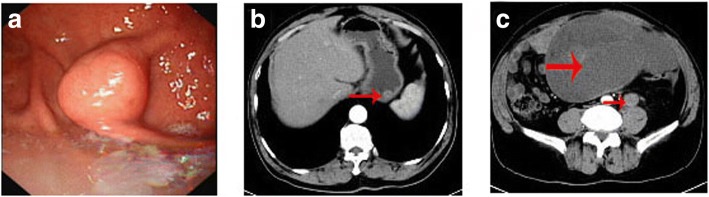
a Upper gastrointestinal (GI) endoscopy showed a tumor arising from the greater curvature of gastric body and extending into the lumen. b CT scan showed a marked enhancement of polypoid mass protruding into the gastric lumen. c CT scan obtained at a lower level displayed a huge, well-circumscribed, lobulated, intro-abdominal mass and a heterogeneous, round-like mass close to the left psoas
